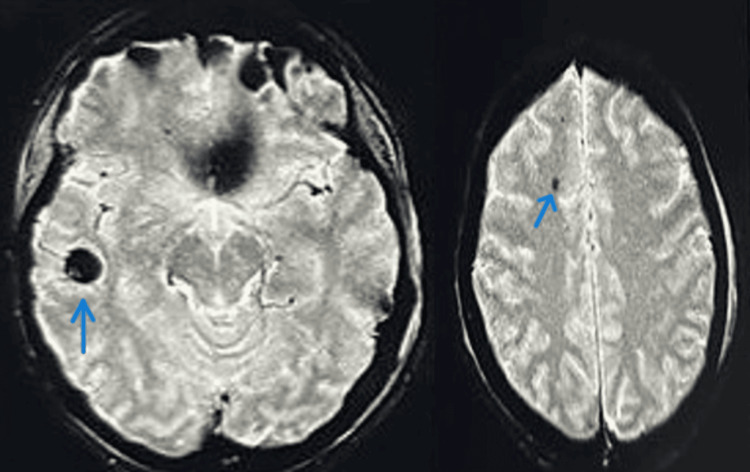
MRI brain: arrows showing hypodense lesions in the cerebral hemisphere due to fat embolism.
